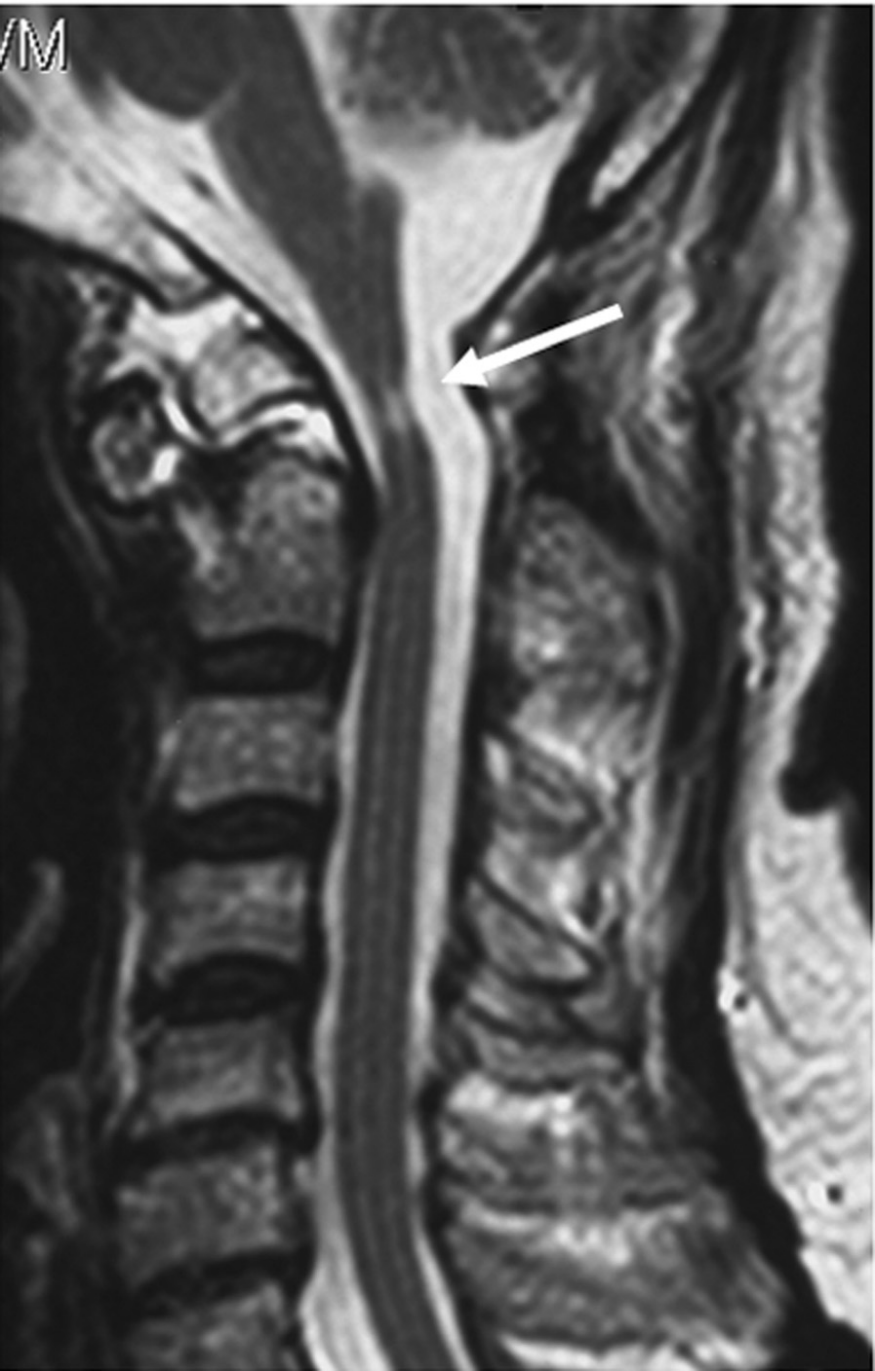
Preoperative sagittal T2-weighted magnetic resonance imaging. Atrophy of the spinal cord with myelomalacia, which was observed as a high intensity lesion (arrow), was seen.
